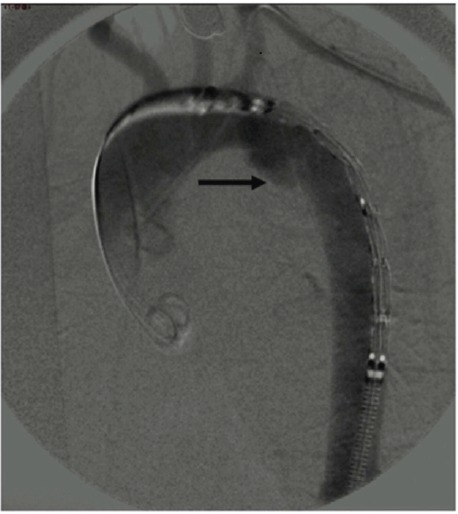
Transesophageal echocardiography, which could be used to guide the procedure, to reduce the amount of injected contrast and to rule out other aortic injuries, was not available in that emergency setting. Therefore, the stent graft was implanted inside the thoracic aorta, close to the origin of the left subclavian artery, under fluoroscopic monitoring and with mean arterial pressure less than 70 mmHg.
